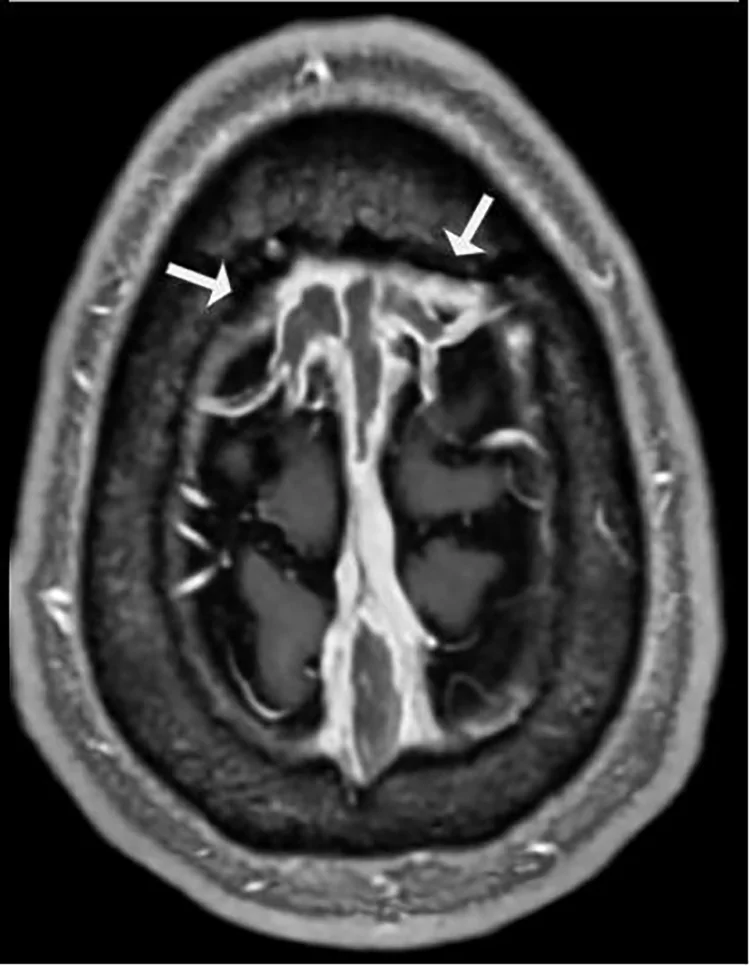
Contrast-enhanced T1-weighted MRI demonstrates filling defects delineating thrombus in the superior sagittal sinus extending into the bilateral bridging veins (arrows)
